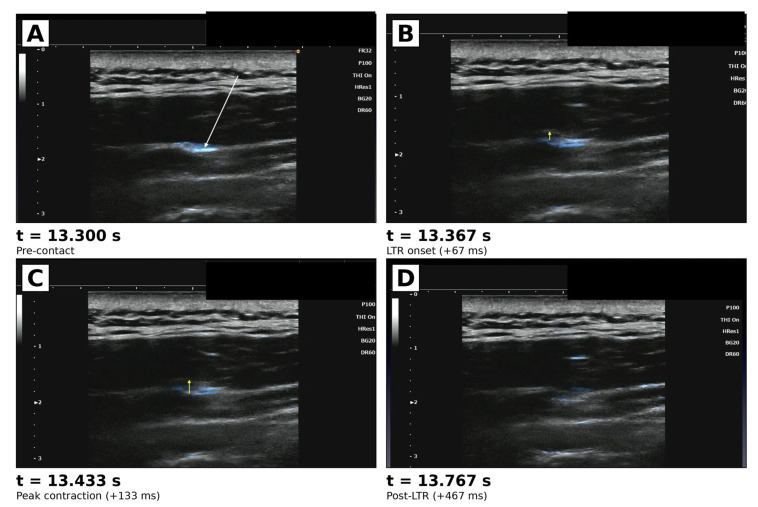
Frame-by-frame ultrasound visualization of a local twitch response (LTR) during ultrasound-guided fascial hydrorelease (FHR), recorded in the trapezius/rhomboid region. (A) Pre-contact (t = 13.300 s): the 27-gauge needle tip approaches the stacking fascia. The needle itself is not directly visible; needle-tip position is inferred from the characteristic tissue motion induced by needle advancement and by small-volume saline injection, visualized via Simple Needle Visualization™ (SNV; Konica Minolta)—a proprietary multi-frame motion-detection algorithm that overlays needle and injectate motion as a blue color signal on the underlying B-mode image (cf. [20,19]). (B) LTR onset (t = 13.367 s; +67 ms): the LTR begins at the moment the needle tip contacts the stacking fascia. The yellow arrow indicates the direction of the upward contraction. (C) Peak contraction (t = 13.433 s; +133 ms): maximum tissue displacement during the LTR. Contraction involves the stacking fascia and adjacent muscle. (D) Post-LTR (t = 13.767 s; +467 ms): tissues return to a relaxed state. The saline spread appears more dispersed than in (A), reflecting tissue displacement during contraction. In the present retrospective cohort of 11,205 ultrasound videos recorded during FHR, 100% of 89 final primary analysis local twitches (after independent dual-rater determination with adjudicated consensus) were generated while the 27-gauge needle tip lay within stacking fascia, whereas no local twitches were observed when the needle tip lay outside stacking fascia in the primary cohort. Local twitch is a brief (less than 1 s) involuntary contraction observed at the moment the needle tip contacts the stacking fascia, involving the stacking fascia and adjacent muscle. SNV is not a Doppler-based modality and therefore does not have an associated pulse repetition frequency, velocity scale, or color-Doppler mechanical-index setting; it operates by frame-to-frame motion analysis distinguishing moving (needle/injectate) from stationary (tissue) image components [19].
